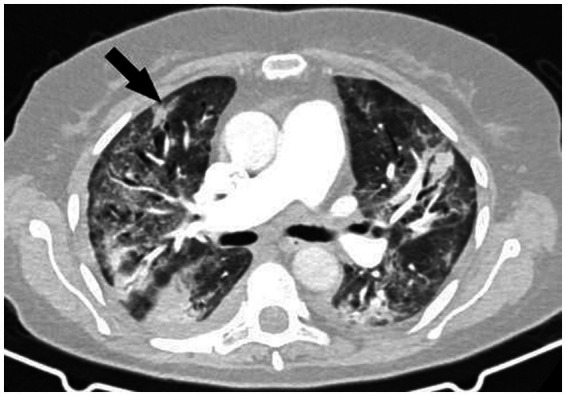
The CT scan shows wedge-shaped and segmental or lobar consolidation.
